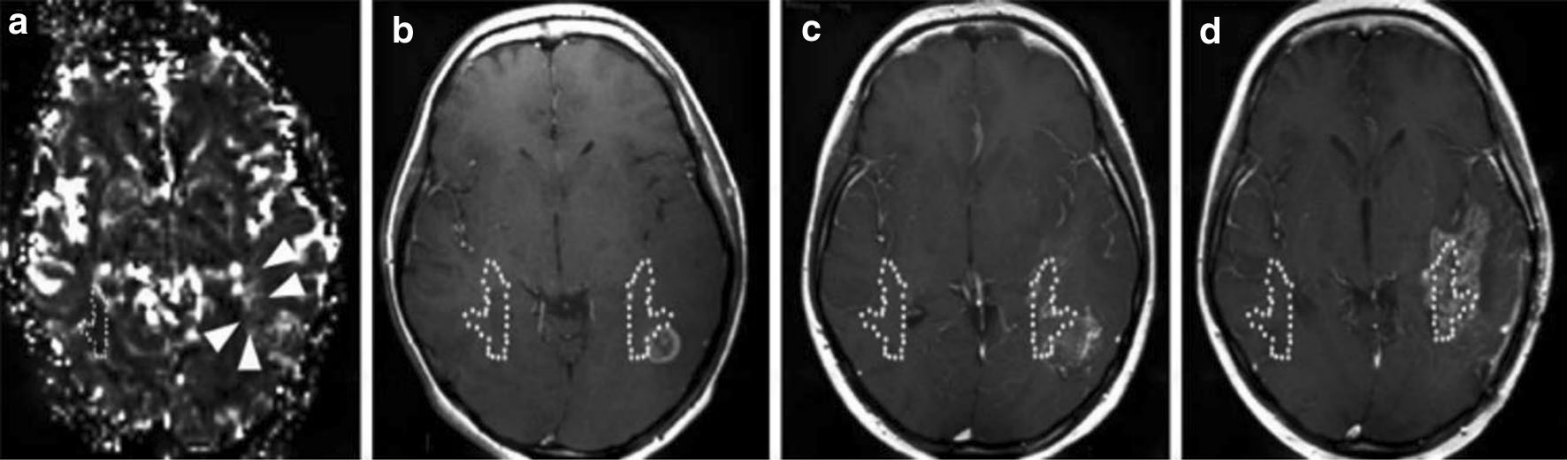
On rCBV map: a a specific directional stripe-like pattern that extended beyond the contrast-enhancing tumour rim, was visible (white arrows). Contrast-enhanced T1-weighted imaging of the baseline MR scan (b), and the 3 (c) and the 6 months (d) follow-up MR scan show that this extension (yellow delineation) was covered with contrast enhancement in its entirety after 6 months. The delineation on the contralateral side marks a contralateral reference (reprinted with permission from Blasel et al*.* [48])
